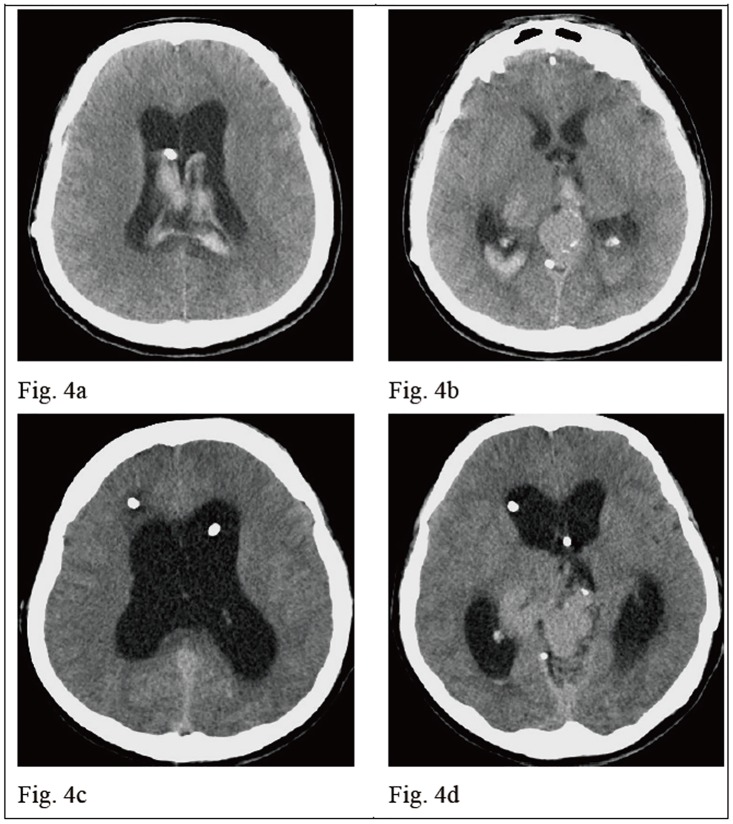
The change of brain CT scans in an intraventricular hemorrhage with hydrocephalus patient (case 2) in 7 days. Fig 4a. and 4b show that the initial brain CT revealed a thalamic vascular lesion and intraventricular hemorrhage in enlarged bilateral ventricles. A right VP shunt tube was placed in the right frontal horn. Fig 4c. and 4d show that the follow-up brain CT on POD 7 revealed a stationary thalamic vascular lesion and regression of intraventricular hemorrhage. Hydrocephalus was persistent and two EVD tubes were placed in the bilateral frontal horns.
